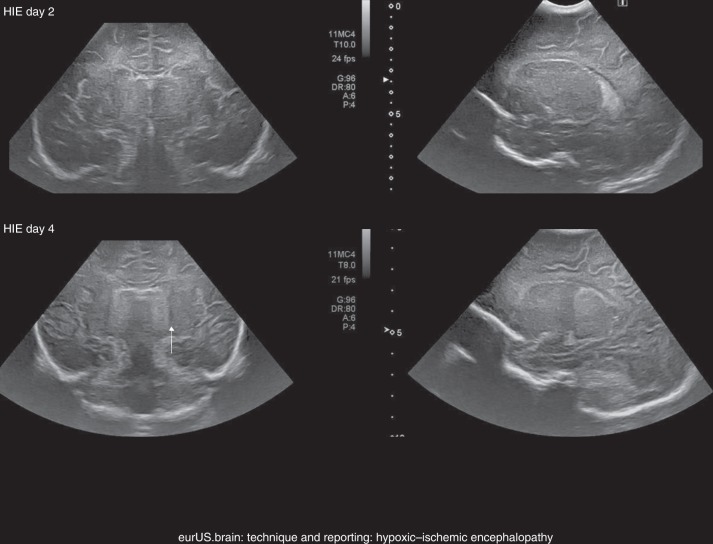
Technique and reporting: hypoxic–ischemic encephalopathy. Intrapartum asphyxia. Term infant, born at 41 weeks’ gestation with asphyxia and hypoxic–ischemic encephalopathy, treated with hypothermia. a, b Ultrasound on admission showing subtle increased echogenicity of the thalami on the coronal (a) but not on the sagittal (b) images. c, d Three days later, there is clearly abnormal increased echogenicity of the thalami in both planes (arrows), which are separated from more mildly echogenic basal ganglia by a band of low echogenicity, representing the posterior limb of the internal capsule (arrowhead).
